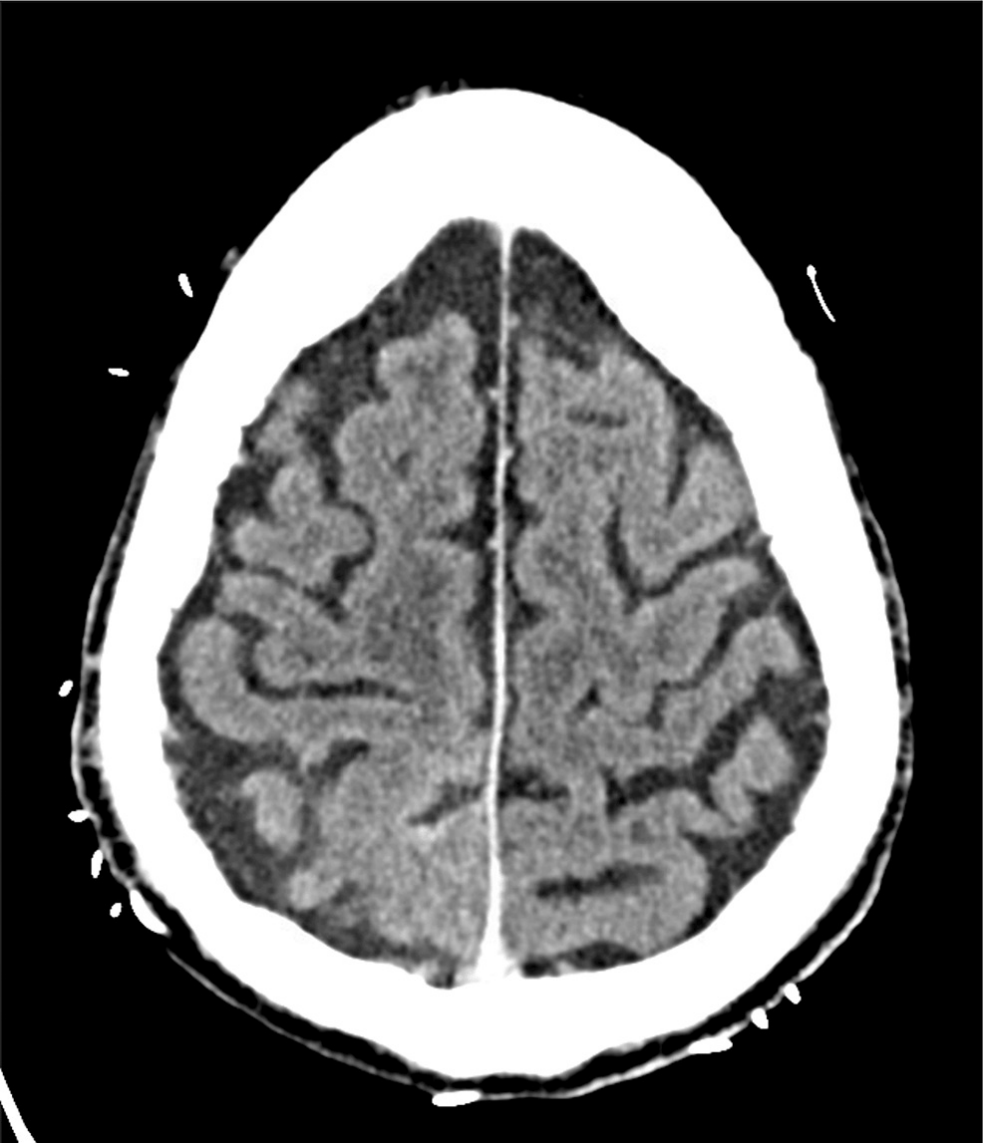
CT head with contrast; subtle contrast enhancement of right parasagittal leptomeninges CT: computed tomography
